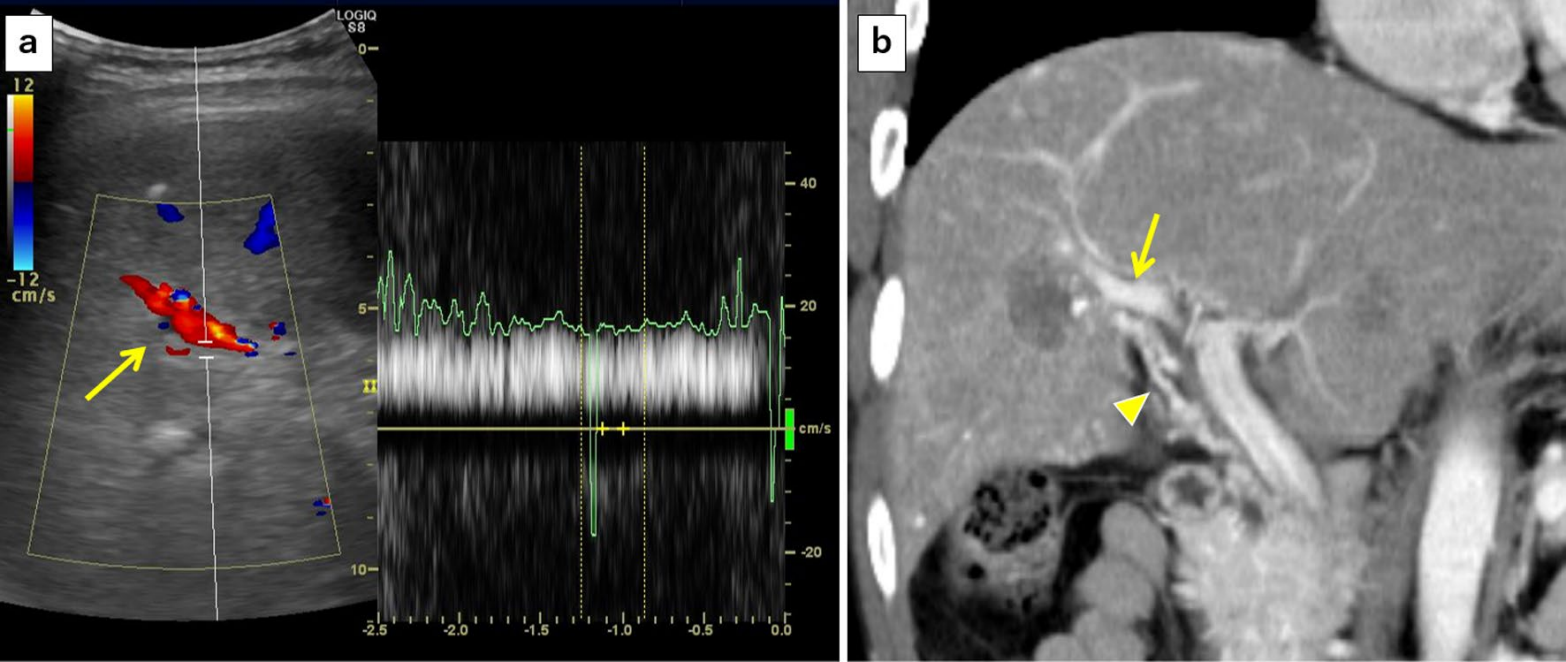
Abdominal ultrasonography (a) and contrast-enhanced CT (b) showed resumption of right portal venous blood flow (yellow arrow) via newly formed collateral vessels (yellow arrowhead) around the right Glissonean pedicle
